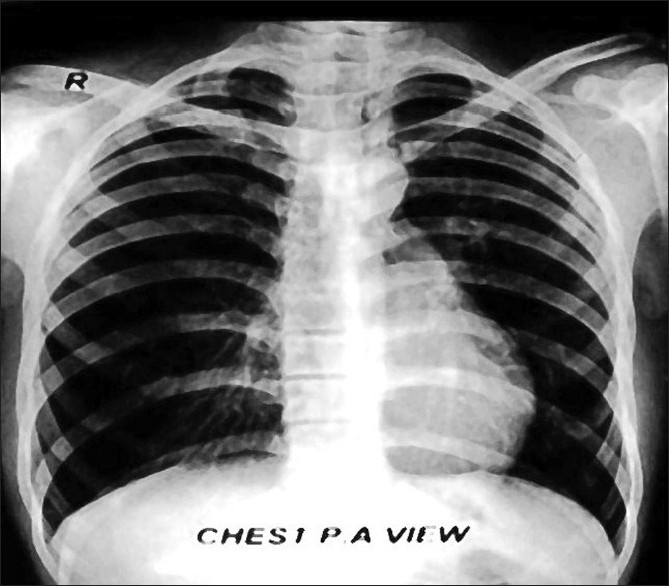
Chest X-ray showing mediastinal shift to left, crowded vascular markings along right paracardiac area and attenuated vascular markings in upper and mid zones of right lung
